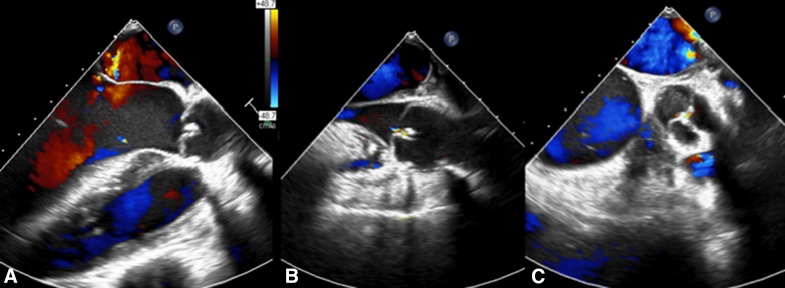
Postoperative echocardiogram demonstrated mild aortic regurgitation and good leaflet coaptation in long-axis view during early diastole (A), late diastole (B) and in short-axis view (C).
